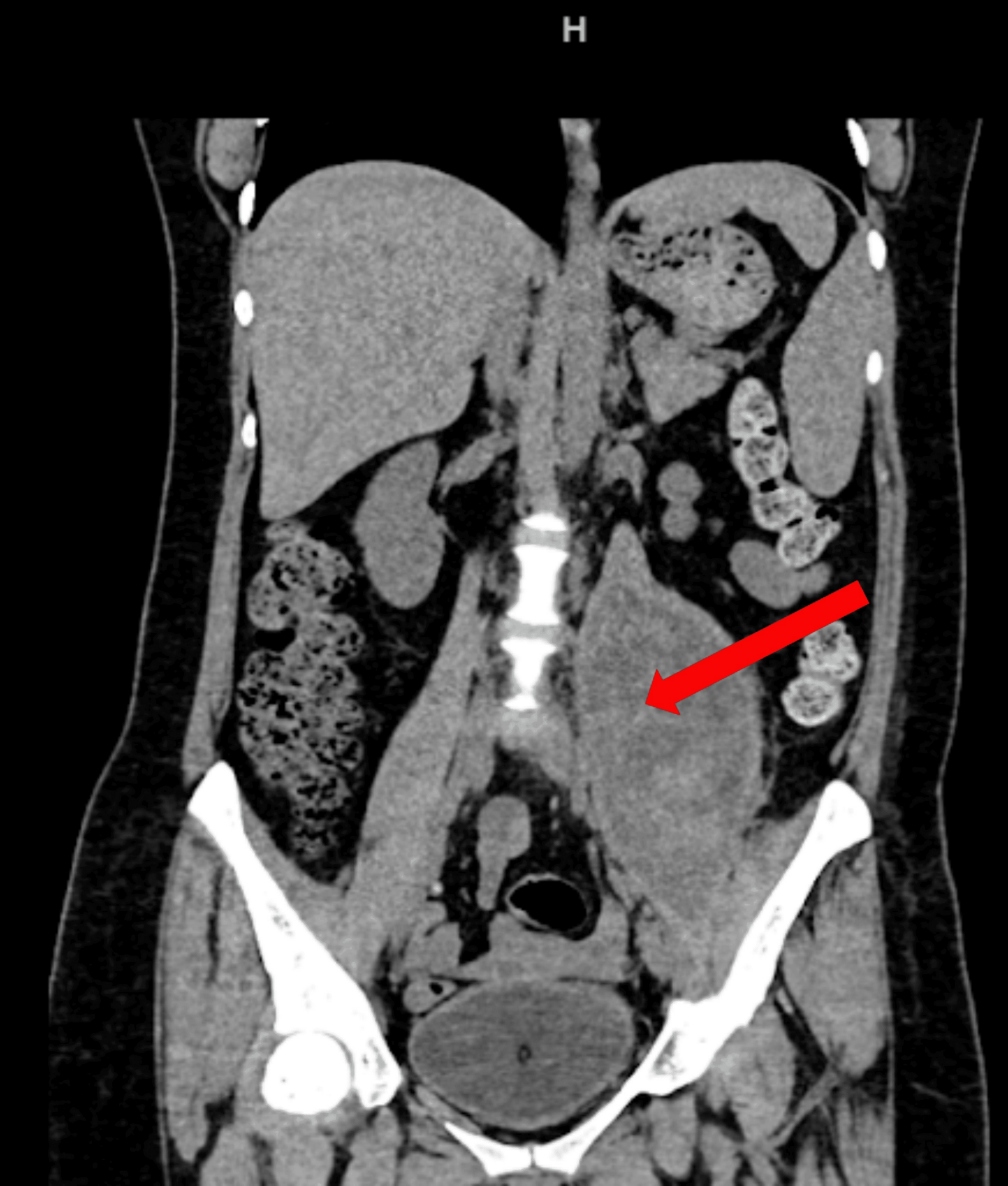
The second CT coronal reconstruction shows a decrease in the hematoma within the left iliopsoas muscle, with an estimated volume of 350 cc.
